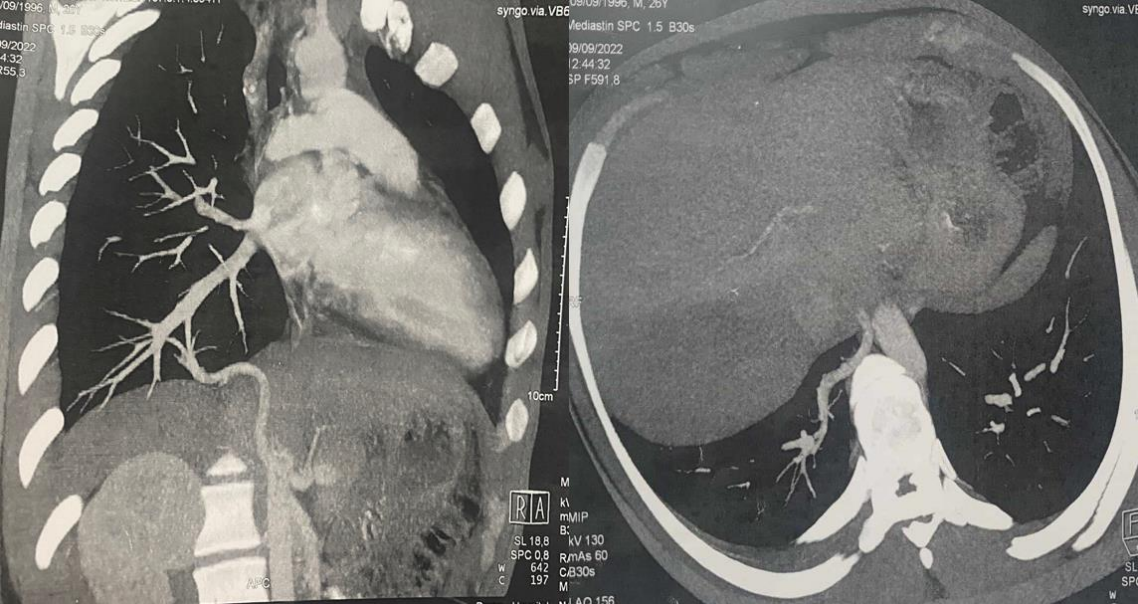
CT angiography showing a 13 mm right posterobasal nodule vascularized by an arterial vessel arising from the subdiaphragmatic aorta above the origin of the celiac trunk
